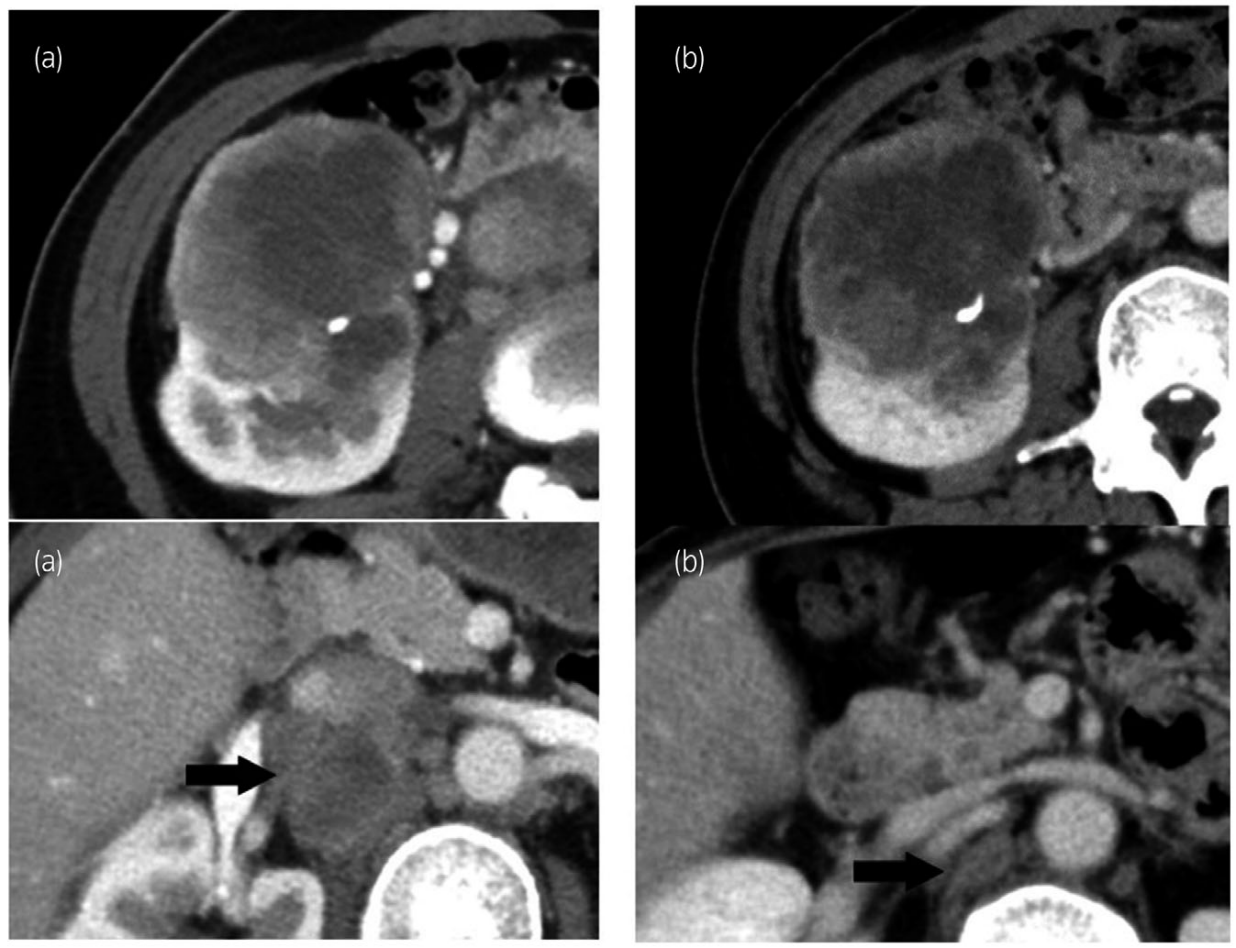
CT of the primary tumor and hilar lymph nodes before (a) and after (b) sunitinib treatment. Arrows indicate metastases. The metastatic lymph nodes have shrunk significantly.
